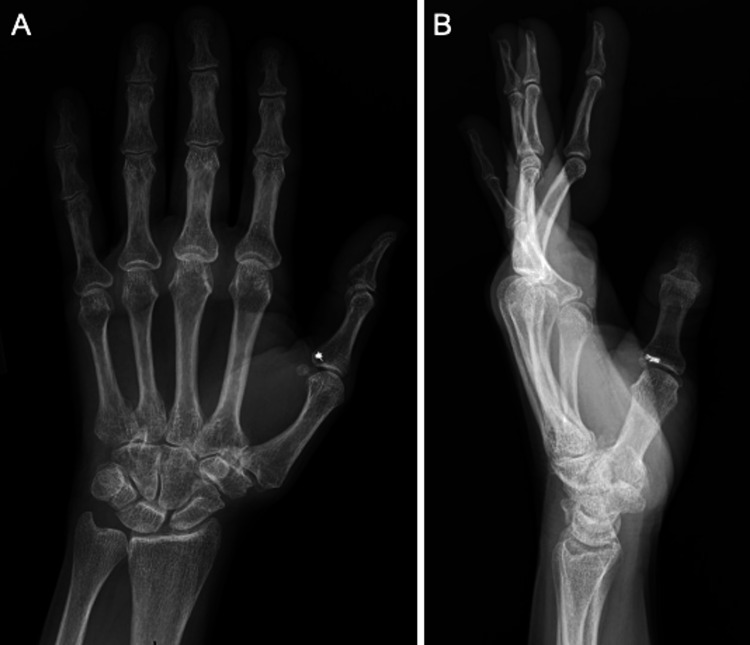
Posteroanterior and lateral radiographs of the hand (A and B) posteroanterior and lateral x-ray views of the left hand. On attempting extension, note extension lag on the lateral view of the long finger at the metacarpophalangeal joint, with hyperextension of the proximal interphalangeal joint. There was no significant long finger interphalangeal osteoarthritis. Thumb with retained hardware from remote ulnocollateral metacarpophalangeal ligament repair.
